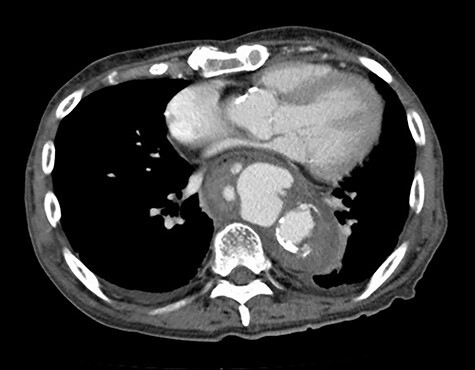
CT at the time of admission shows irregular shape of the aneurysm.
